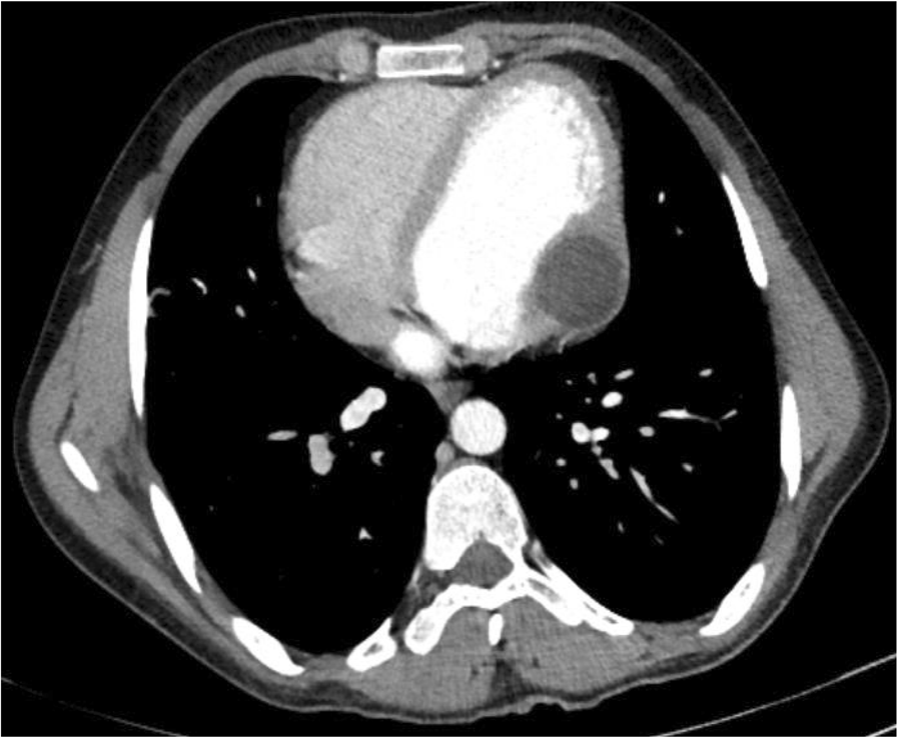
Contrast enhanced CT axial section through the heart: The cystic lesion in the heart is located in the lateral wall of the left ventricle
